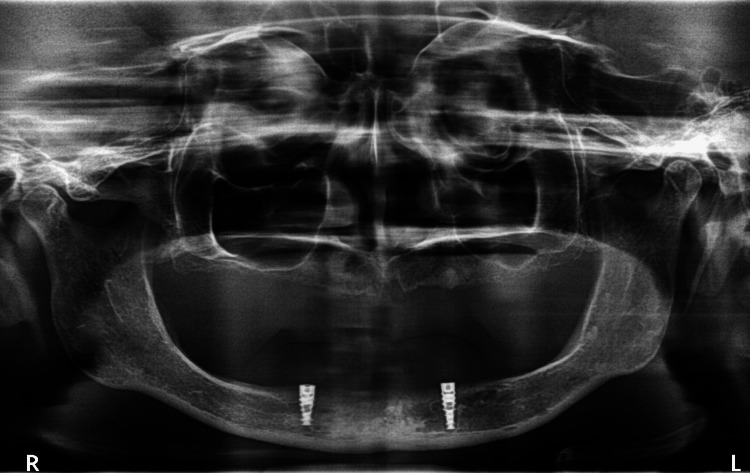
Postoperative orthopantomograph showing the placement of implants. Image Credit: Sourabh Khandelwal
